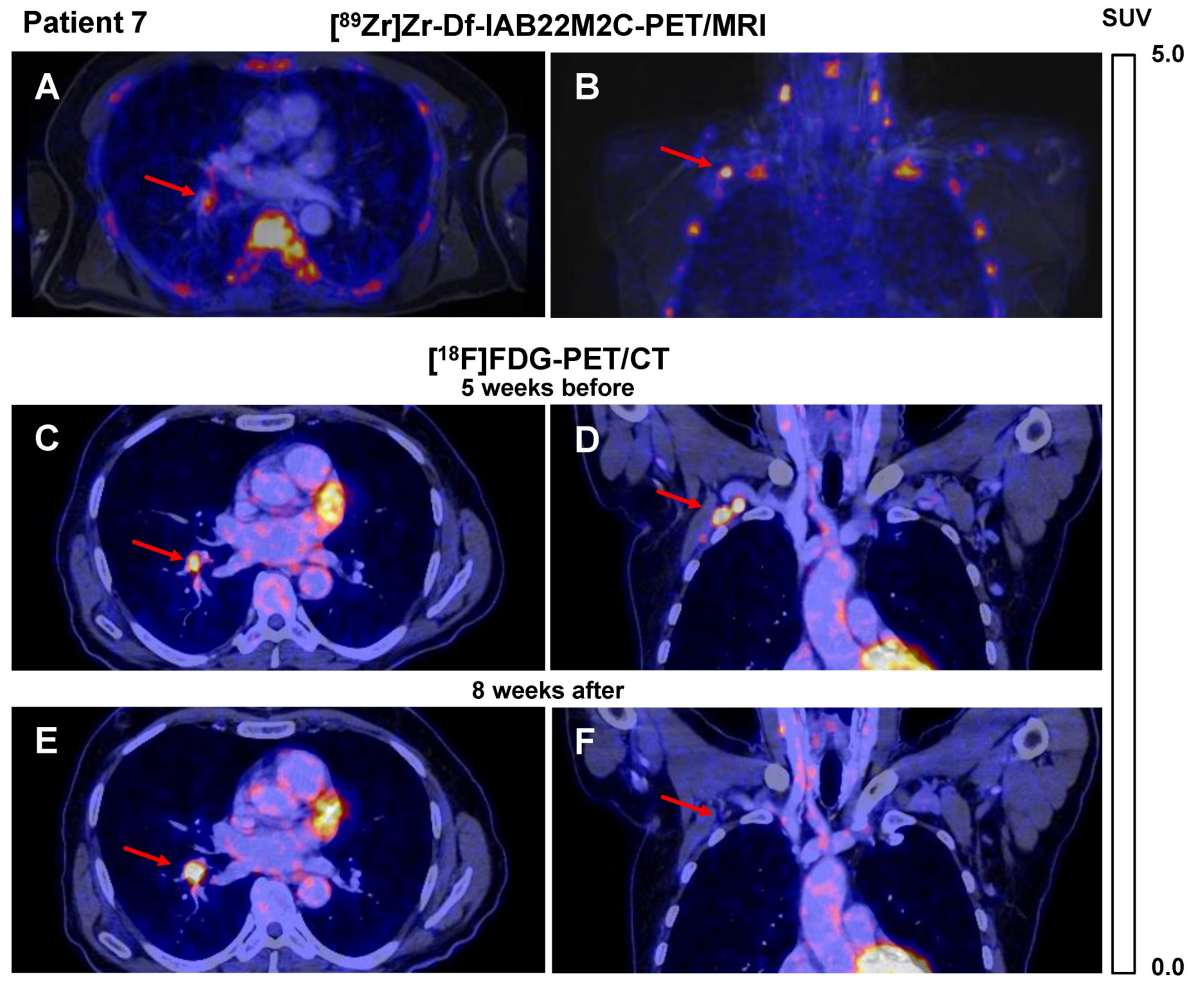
A/B: Axial (A) and coronal (B) fused [^89^Zr]Zr-Df-IAB22M2C PET/MRI of Patient 7 after 4 cycles of combined ICT using ipilimumab and nivolumab until 6 weeks before the scan indicated only faint tracer uptake in a hilar lymph node metastasis (A), intense tracer uptake was found in an axillary lymph node metastasis (B). C/D: Axial (C) and coronal (D) fused [^18^F]FDG PET/CT images of Patient 7 five weeks before [^89^Zr]Zr-Df-IAB22M2C PET/MRI indicated an intense glucose uptake of both the axillary and the hilar lymph node metastasis; these were considered to be viable tumor tissue. E/F: Eight weeks after [^89^Zr]Zr-Df-IAB22M2C PET/MRI, the follow-up [^18^F]FDG PET/CT of Patient 7 revealed a decrease in axillary lymph node metastasis during ICT (F), while hilar lymph node metastasis (E) increased in size and [^18^F]FDG uptake during ICT monotherapy with nivolumab.
